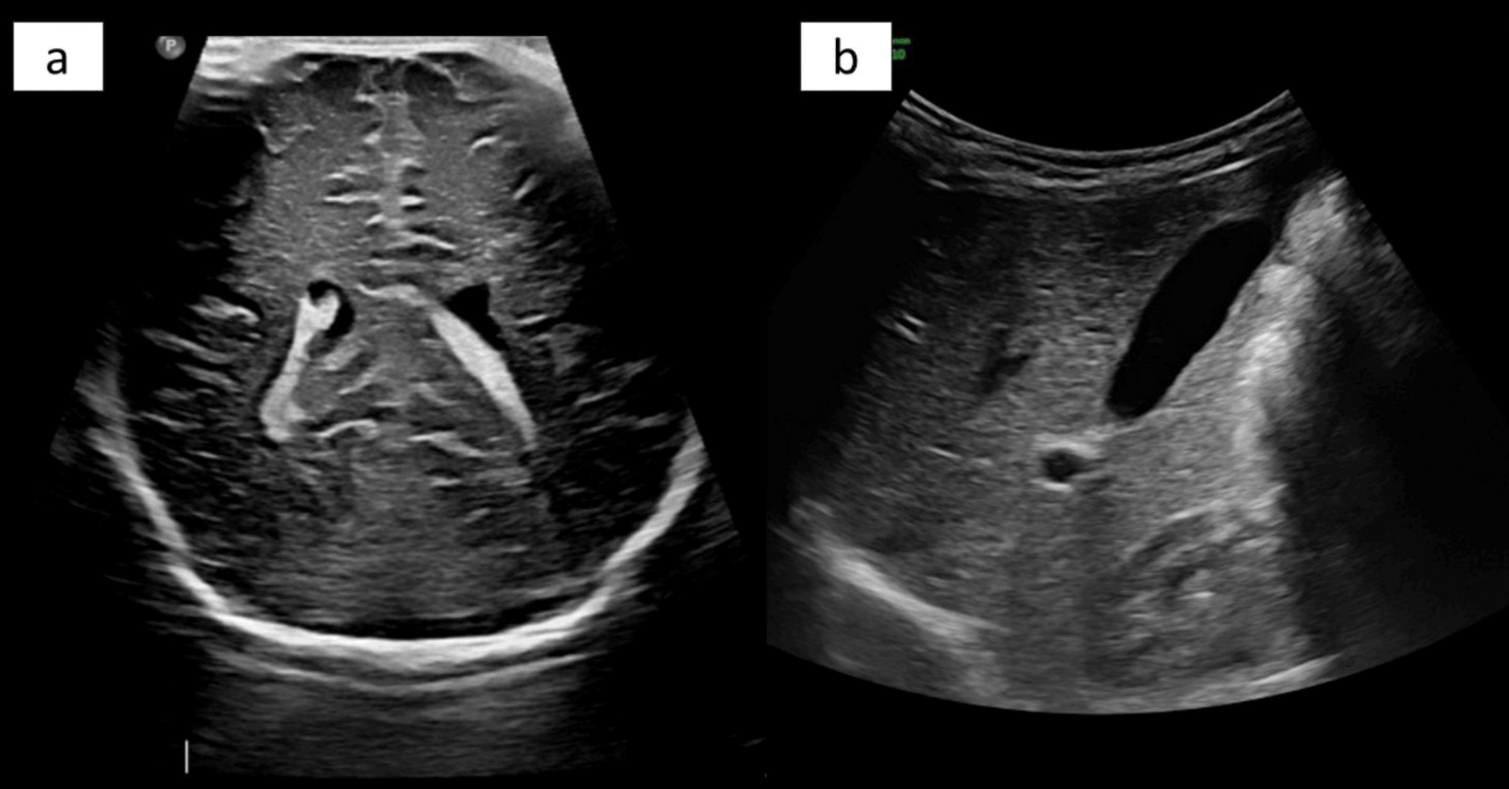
(a) Normal ultrasound of the cranium and (b) ultrasound of the liver of the index case.
